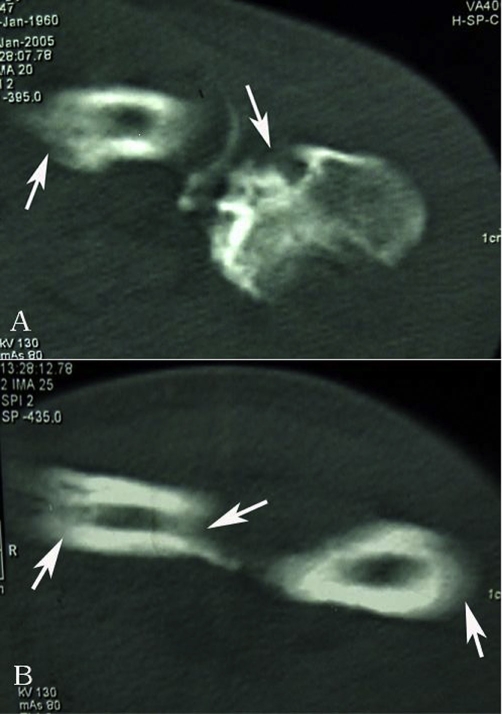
(A) CT image demonstrated aggressive periosteal reaction and multiple lytic lesions (*long arrows*) were presented at diaphysis of left ulna and radius. (B) Cortical resorption and lytic destruction (*long arrows*) was obviously noted.
